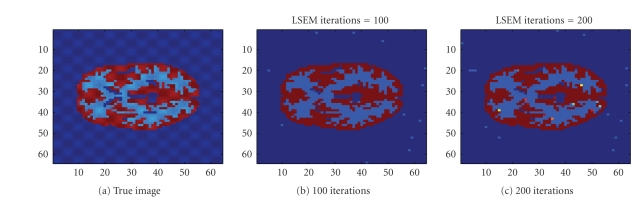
64 × 64 segmented MRI slice of the brain, nonpiecewise constant by adding sin() functions.
